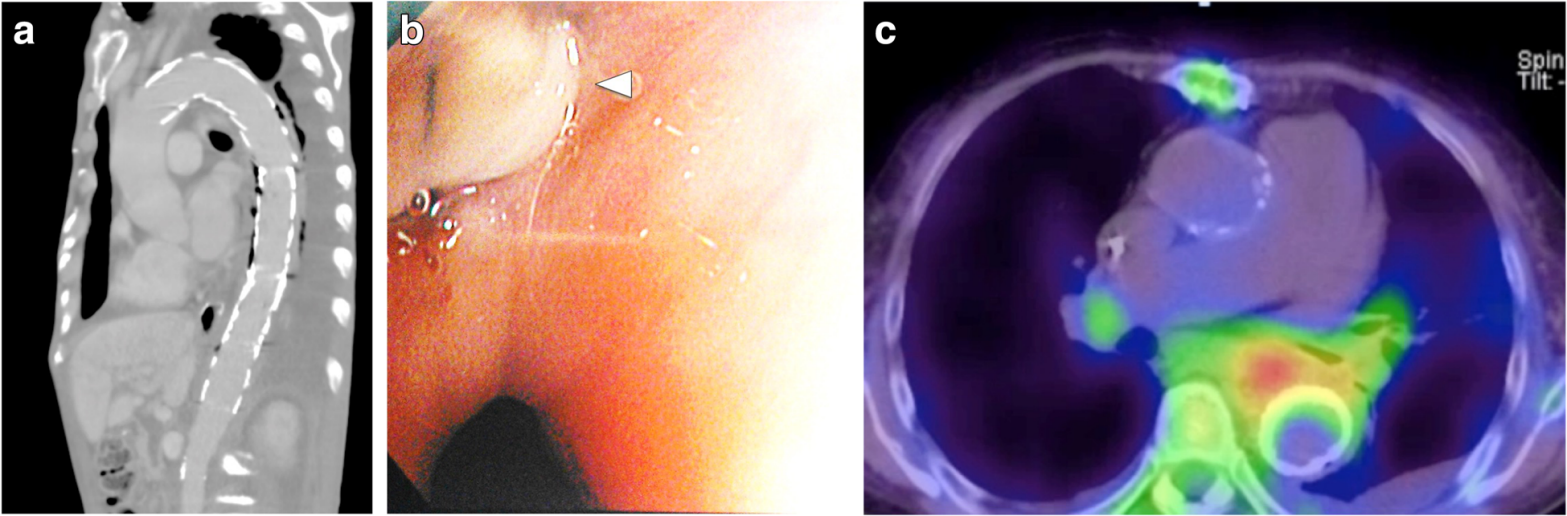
a Preoperative computed tomography scan of a combined repair case demonstrating massive air bubbles around the previous stent-graft. b: Endoscopic view showing exposure of the sutures and pledget (white arrow head) of the previous graft. c: Preoperative gallium scintigraphy demonstrating enhancement between the aorta and esophagus
